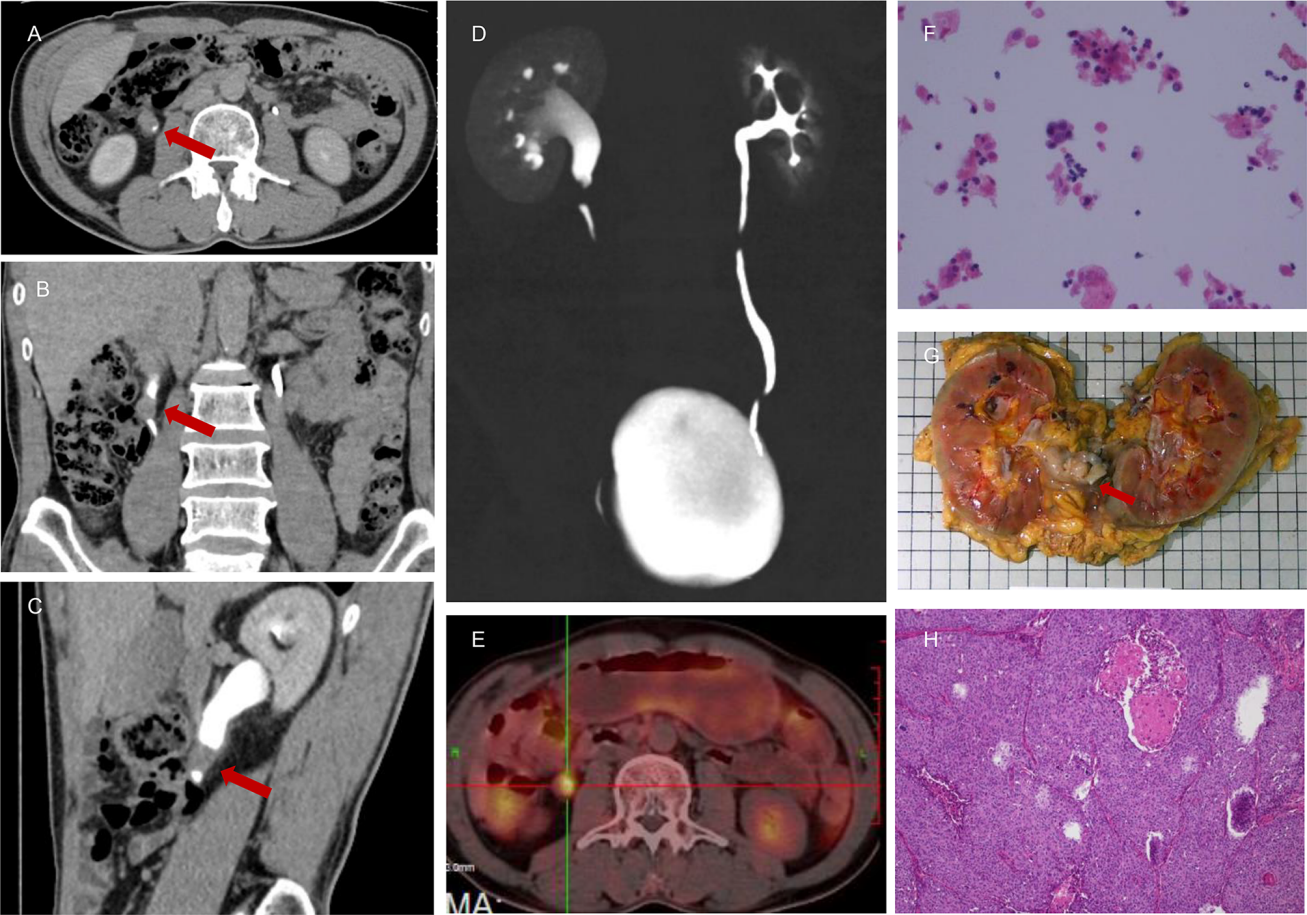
A 55-year-old male presented with persistent painless gross hematuria for 1 month. The results of CTU indicated malignant tumor (1.2 cm * 1.7 cm) of the right ureter (A–D). There were nuclear heterogeneous cells suggested by urine cytology that were regarded as malignant tumor cells (F), and the NLR value was 3.99. Intense 18F-FDG uptake was also noted in the right upper ureter (E), which indicated a high probability of malignant tumor. This patient was subjected to right RNU, and the final pathological report confirmed a diagnosis of right upper ureteral carcinoma (G, H).
